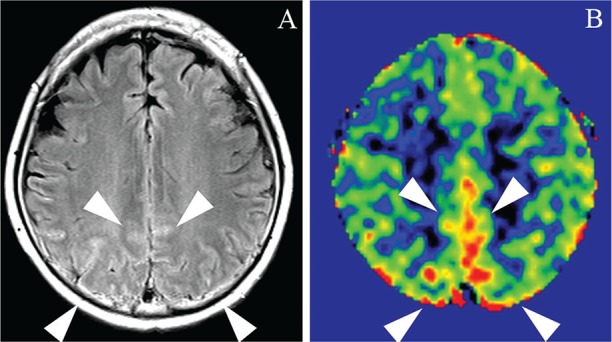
A 63-year-old woman with meningeal involvement due to unspecified viral infection. High intensity along the cerebral sulci is observed on fluid-attenuated inversion recovery (A: arrowheads). High perfusion is prominent in the affected leptomeningeal areas on arterial spin-labeling magnetic resonance imaging (B: arrowheads).
